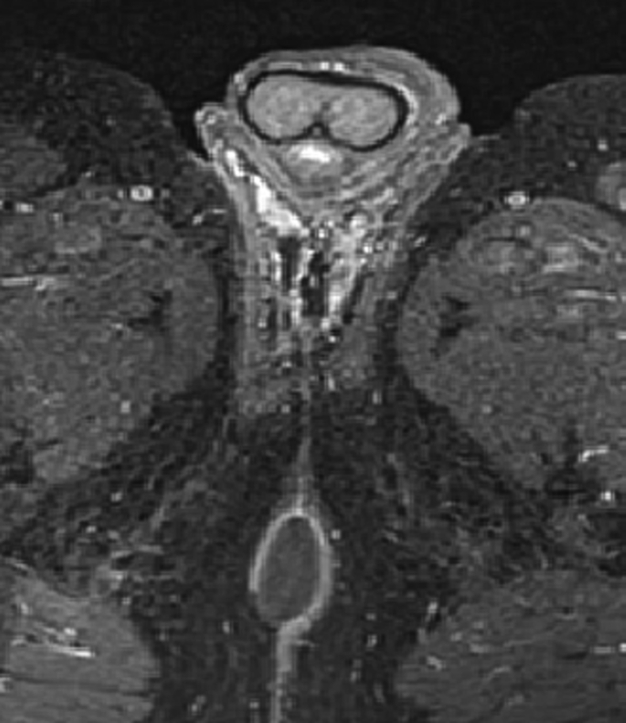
Pelvic MRI images.
